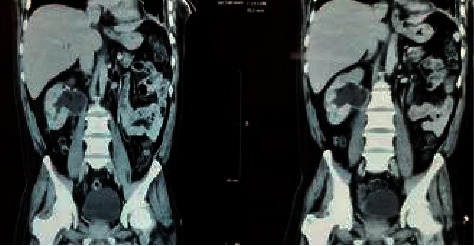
CT abdomen showed right-sided hydronephrosis.
